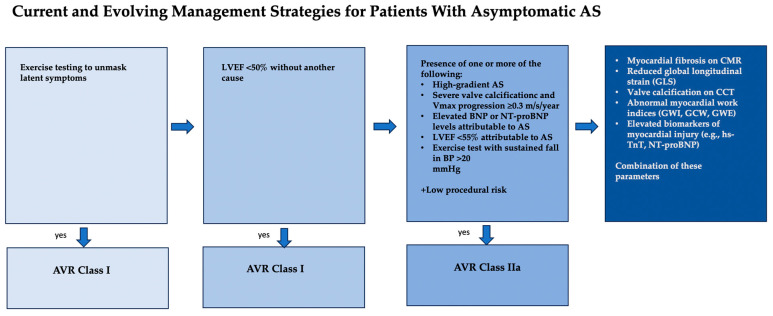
Evaluation and treatment of patients with asymptomatic severe aortic stenosis. Beyond determination of left ventricular ejection fraction and verification of asymptomatic status with exercise testing, there are several established indices of disease severity supported by the American Heart Association/American College of Cardiology (AHA/ACC) and European Society of Cardiology/European Association for Cardio-Thoracic Surgery (ESC/EACTS) guidelines, as well as evolving novel indices. The approach to the asymptomatic patient may include unmasking of symptoms through stress testing, assessment of EF, and evaluation of severity parameters using echocardiography, CT, and stress imaging. Future perspectives involve combining parameters derived from advanced imaging techniques, such as global longitudinal strain (GLS), myocardial work, and myocardial fibrosis on cardiac MRI—with circulating biomarkers, including troponin. AS = aortic stenosis; AVR = aortic valve replacement BNP = B-type Natriuretic Peptide; BP = Blood pressure; CCT = cardiac computed tomography; CMR = cardiac magnetic resonance; hs-TnI = high-sensitivity Troponin I LVEF = left ventricular ejection fraction; GCW = global constructive work; GWI = global work index; GWE = global work efficiency.
